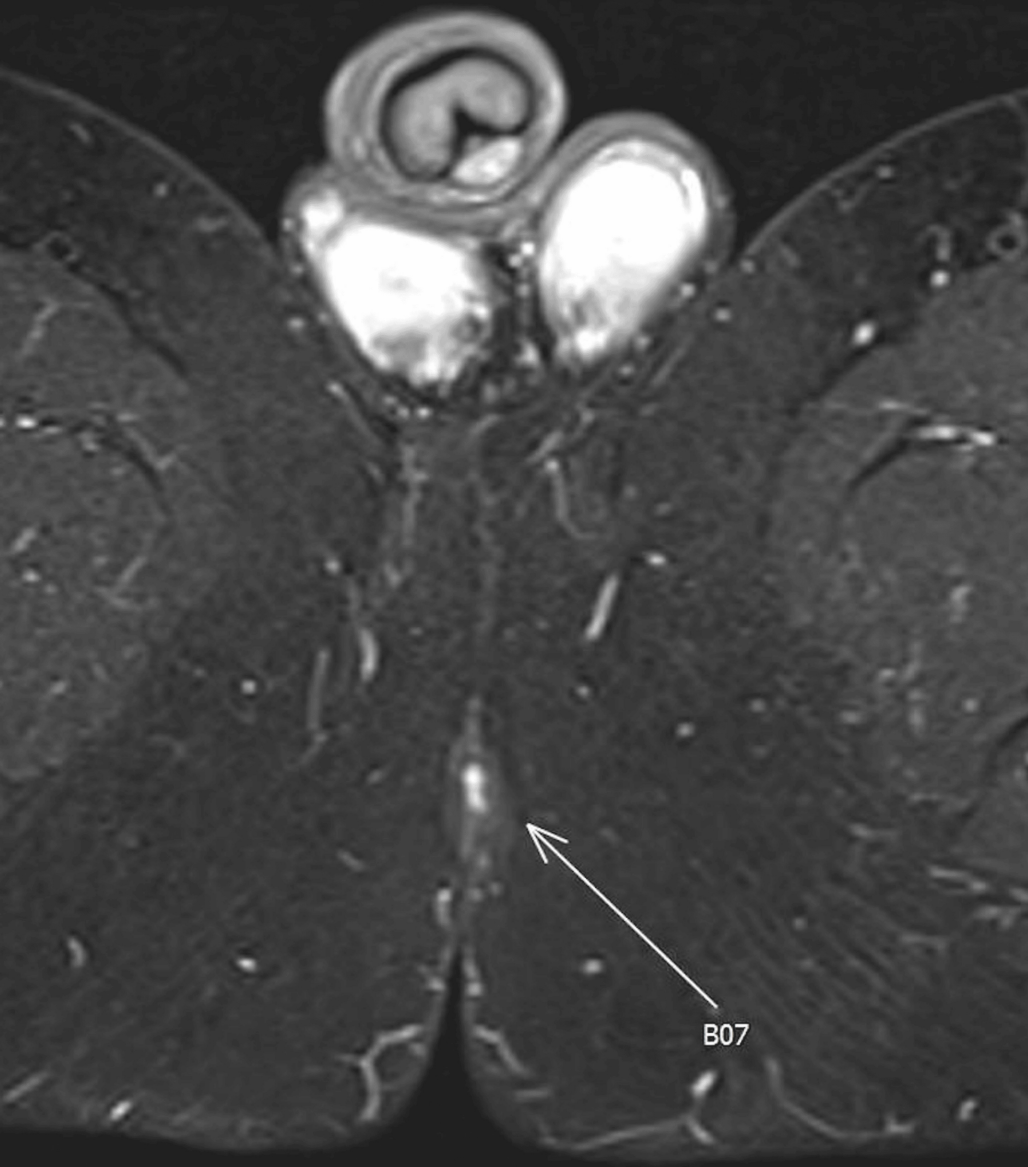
Pelvic MRI showing small sinus tract (arrow) in the perineum midline anterior to the anus
